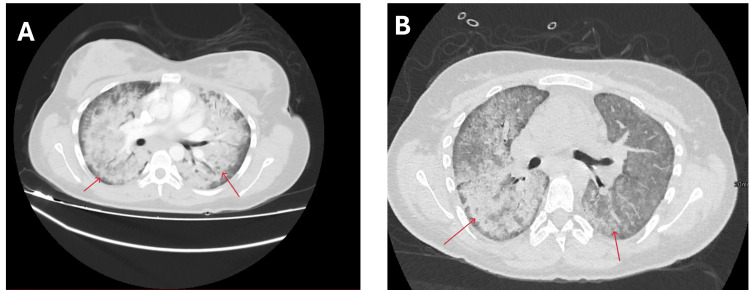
Axial chest CT images. (A) Initial scan at presentation demonstrating diffuse bilateral consolidations and centrilobular nodules, consistent with active diffuse alveolar hemorrhage. (B) Scan during disease relapse, showing recurrence of diffuse alveolar hemorrhage.
